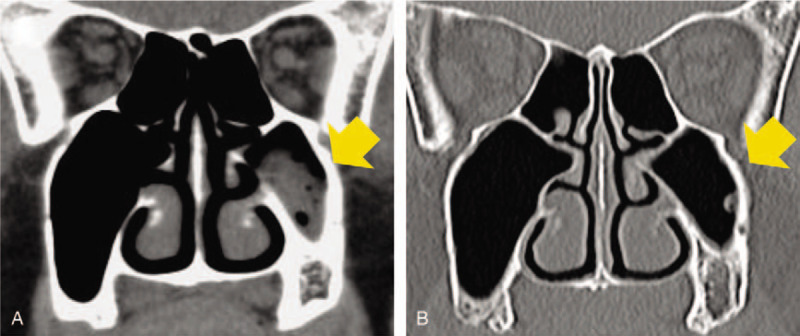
Sinus CT scan of the patient before and after treatment of sinusitis. The mucosal thickening and fluid were found in his left maxillary paranasal sinus (left, arrow), which was substantially improved after medical treatment of sinusitis (right, arrow). CT = computed tomography.
